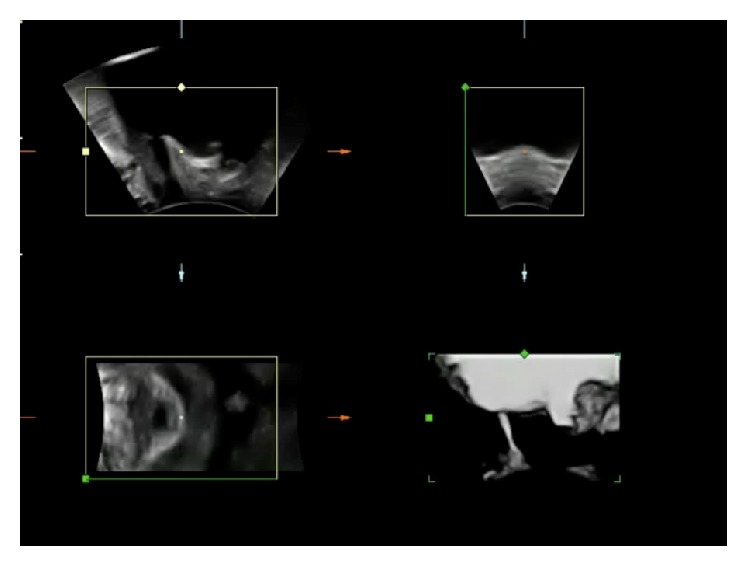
3D/4D transperineal ultrasound image.
